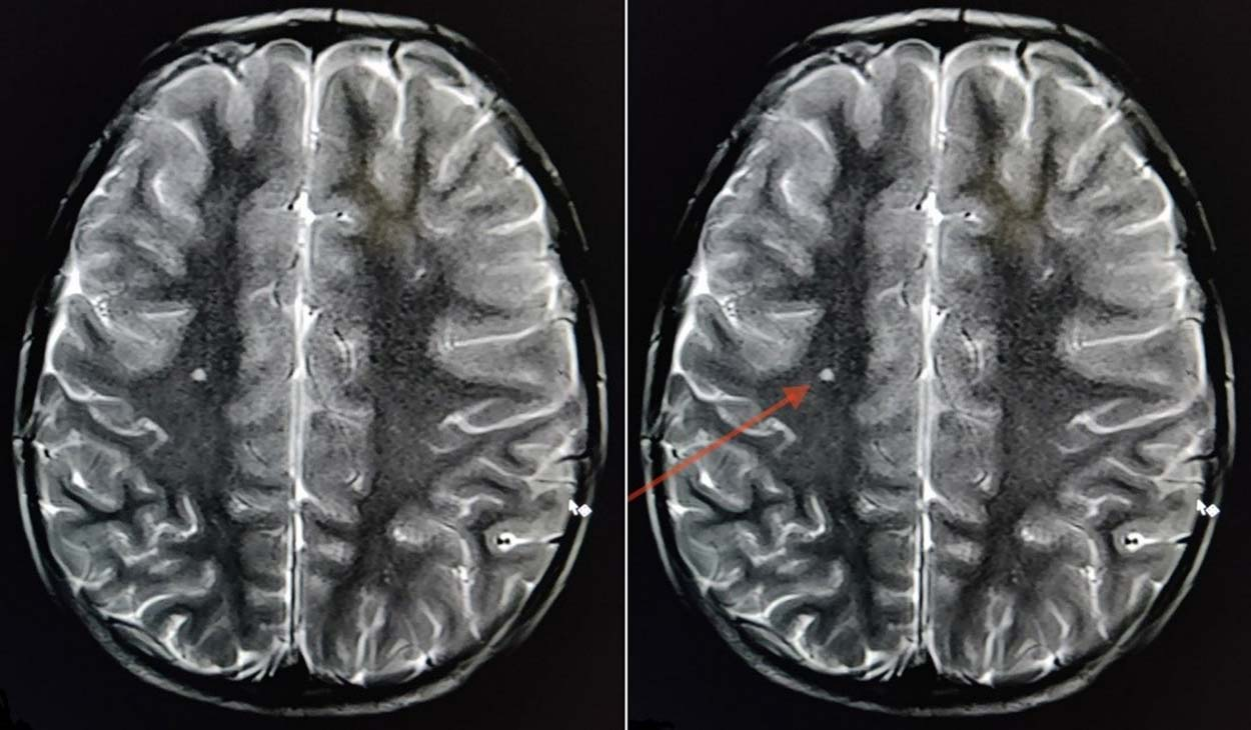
T2/FLAIR high signal intensity foci in right parietal lobe (left unmarked, right marked); features suggestive of non-specific demyelination (red arrow) and rest of the brain showing normal morphology with normal parenchymal signal intensity with no evidence of brain oedema, recent haemorrhage or infarction.
